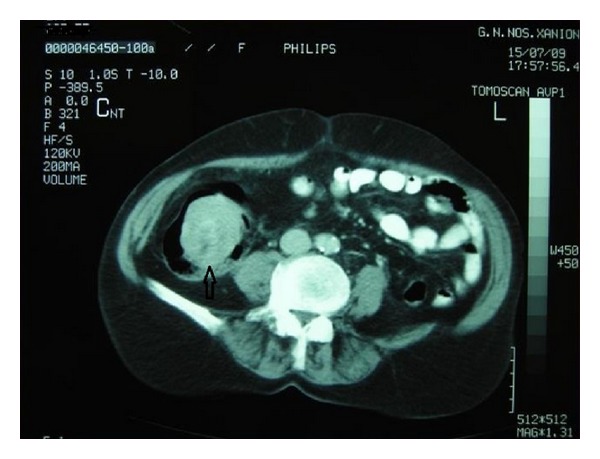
Contrast-enhanced abdominal CT revealing the presence of a mass at the cecum level (arrow).
